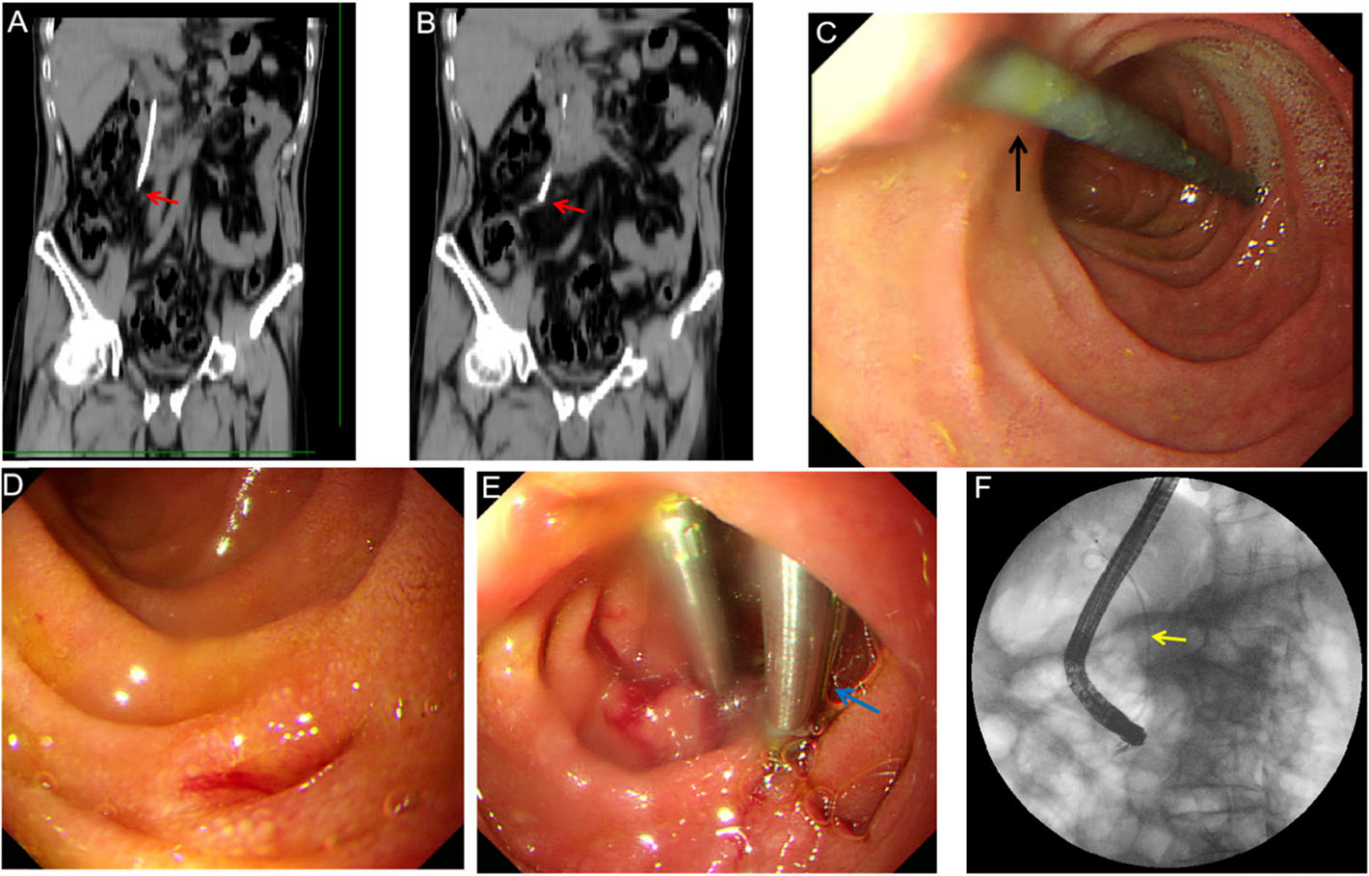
Duodenal perforation due to biliary plastic migration successfully managed by endoscope. a and b Coronal CT scan of the abdomen demonstrated that the biliary plastic stent (red arrow) had migrated and perforated through the duodenal wall. c Distal tip of biliary stent (black arrow) penetrated the duodenal wall which was confirmed during endoscope. d The migrated plastic stent was extracted by snare and perforation was detected during endoscopy. e The defect was successfully closed by hemostatic clips (blue arrow). f An endoscopic nasobiliary drainage (ENBD) tube (yellow arrow) was then inserted
